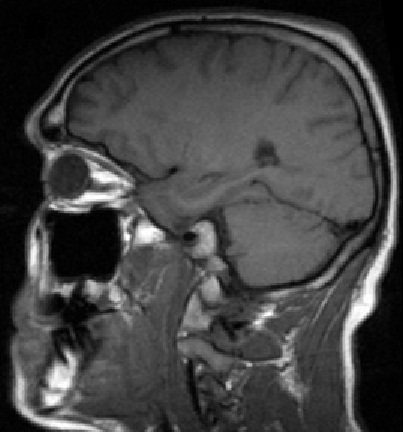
Brain MRI, T-1 sagittal view shows the high signal has completely resolved two years later.
